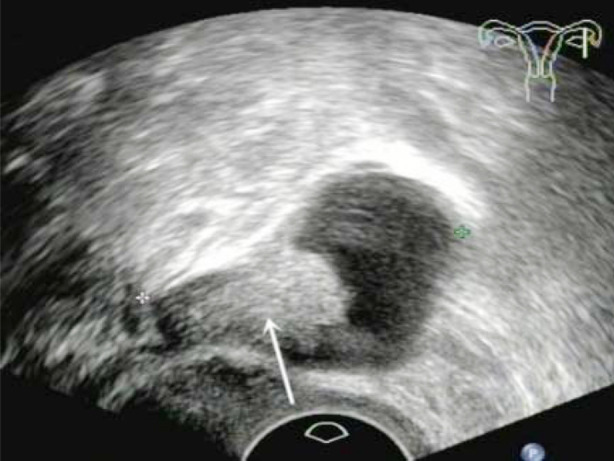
A patient with left type I PFTC (No. 2). The transvaginal two-dimensional ultrasound showed the sausage-shaped cystic mass with papillary nodules in left adnexal region (arrow).
